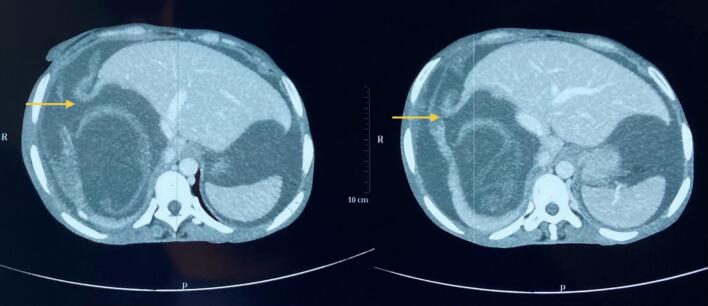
CECT Abdomen: Axial section showing thick-walled cystic lesion in the right lobe of the liver with a defect in the anterosuperior aspect of its wall (yellow arrow). CECT: Contrast Enhanced Computed Tomography. (For interpretation of the references to colour in this figure legend, the reader is referred to the web version of this article.)
